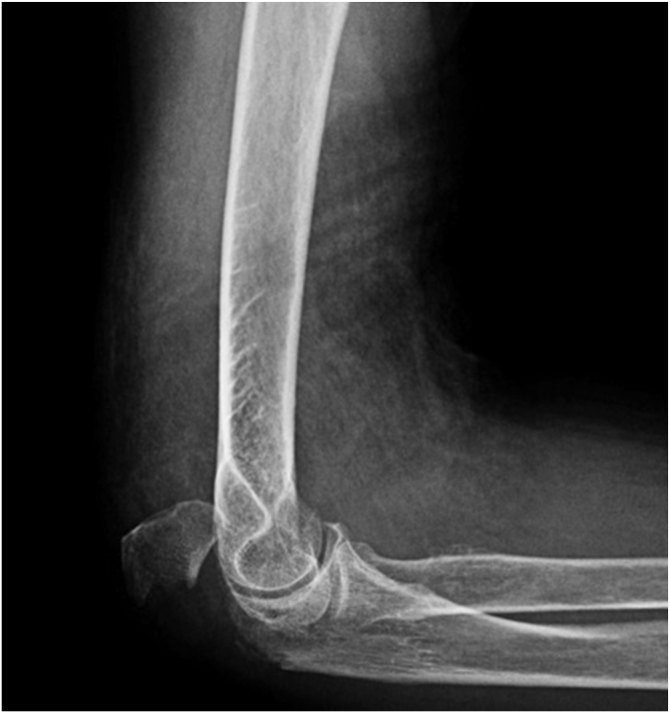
Radiograph of an 82-year-old female with a displaced Mayo type IIa olecranon fracture.
